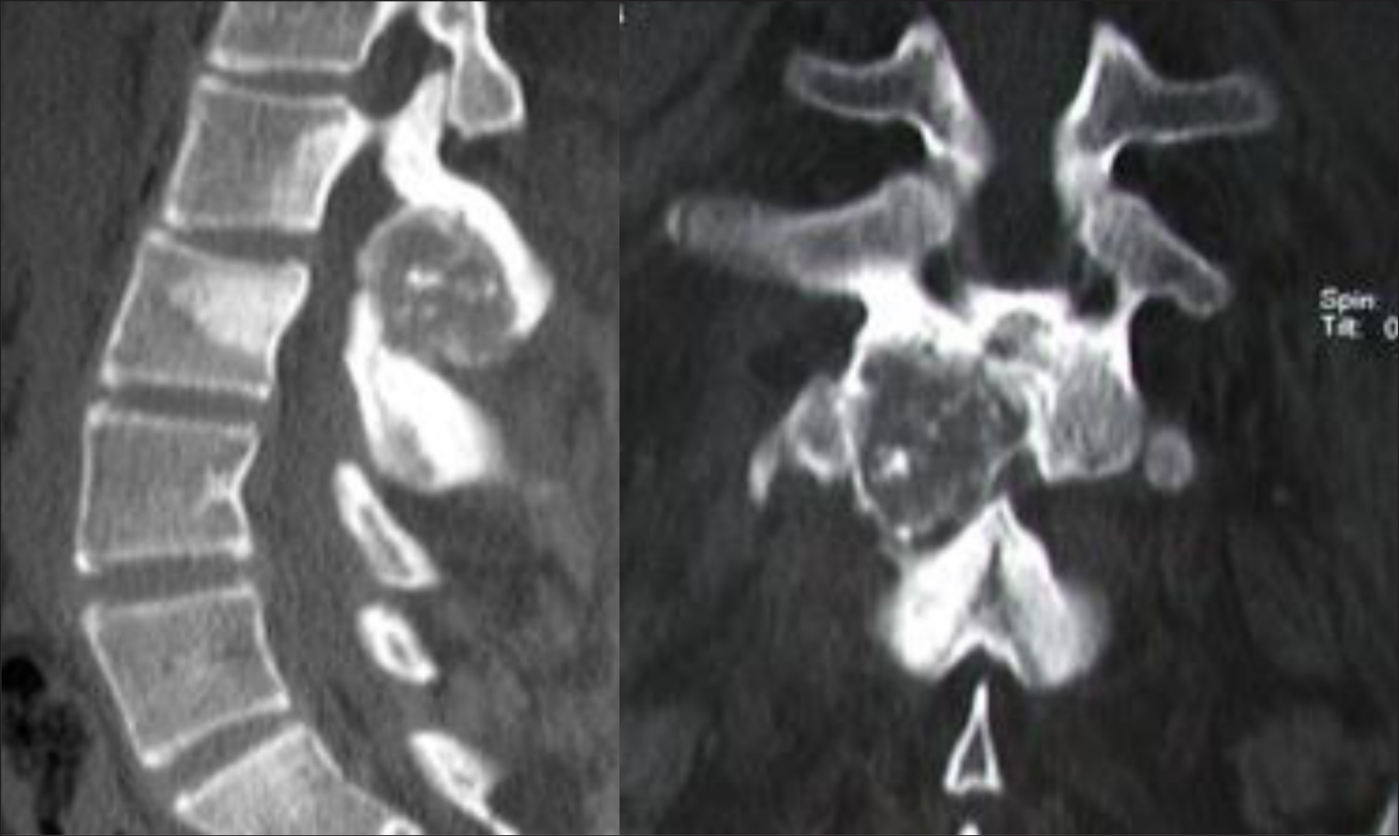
Osteoblastoma of L2. CT scan shows an expansive osteolytic lesion of the L2 neural arch with central calcification, sclerosis of the body and the neural arch of L2 and L3.
